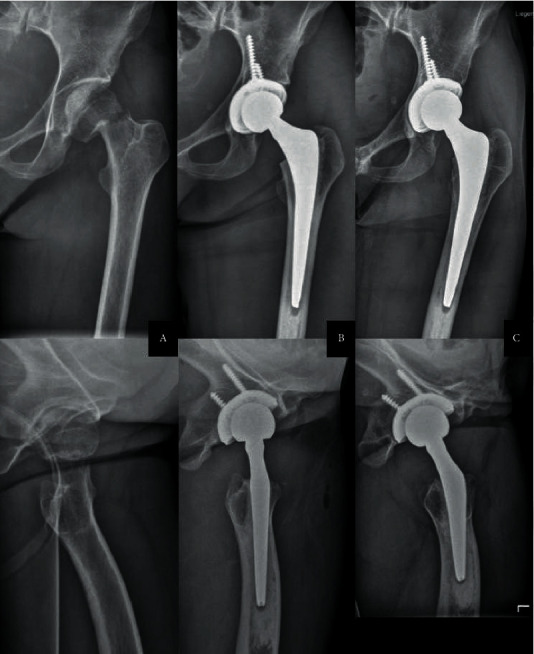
Anteroposterior and axial radiographs of the left hip from case one at the time of the dislocated stress fracture of the femoral neck (a) on the second postoperative day after hybrid THA (b) and at the one-year mark (c) Several months after untreated acute PJI with *S. aureus* the implants are well fixed, in particular, the cup is fully integrated. Note thinning out of the cancellous bone in the proximal femur as well as of the cortical bone on the femoral diaphysis, with an enlarged medullary cavity, indicating osteoporosis. The patient suffered from refractory anorexia nervosa.
